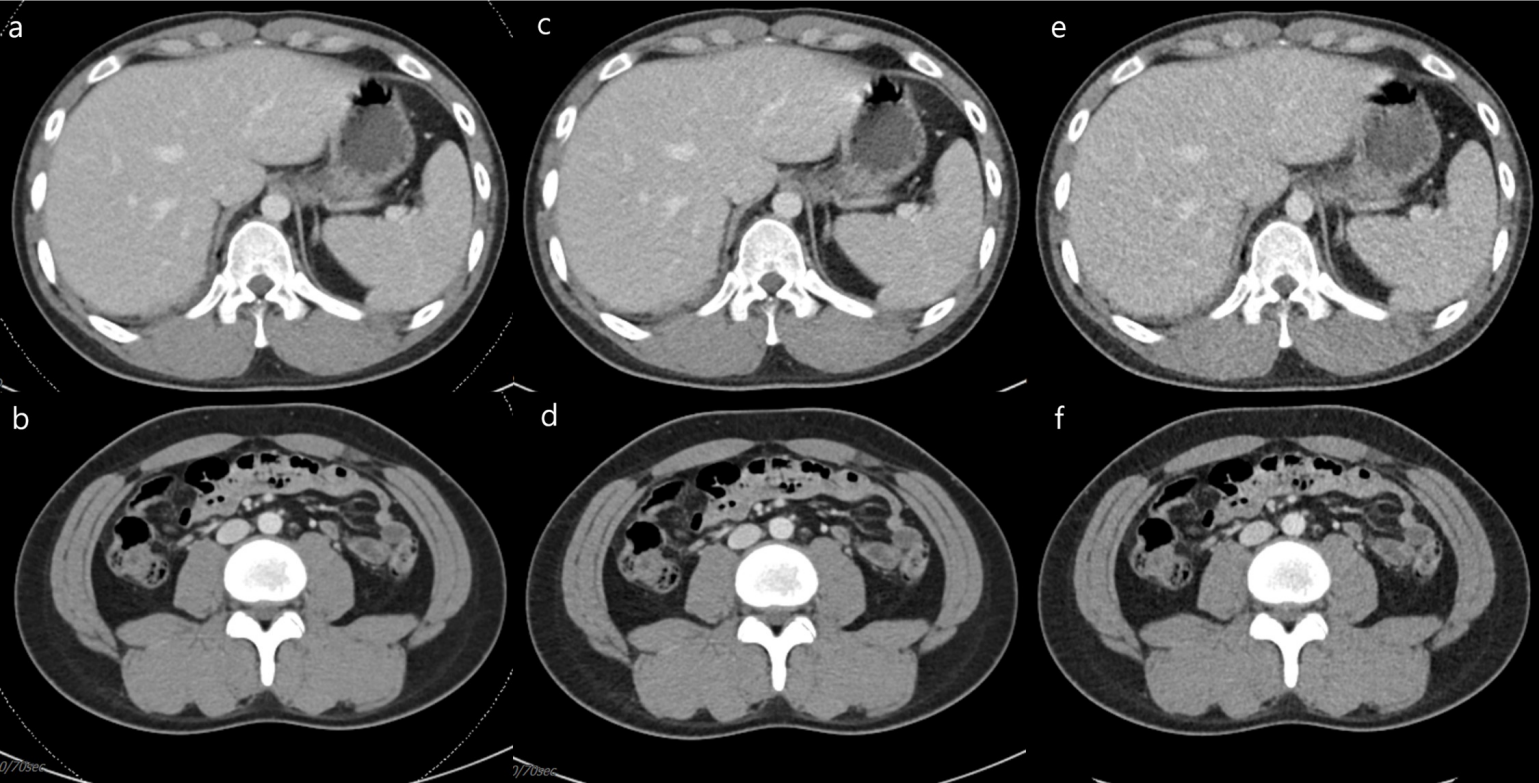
Abdominopelvic computed tomography (CT) images of a 23-year-old woman with Hodgkin’s lymphoma, complete remission state (body mass index, 24.2 kg/m^2^; effective diameter, 24.0 cm). The three different types of CT images, acquired according to the radiation dose (a-b: standard-dose CT, 5.6 mSv; c-d: low-dose CT, 3.7 mSv; e-f: ultralow-dose CT, 1.8 mSv), show the absence of enlarged lymph nodes and splenomegaly. Identical reports of the aforementioned features were also obtained from two radiologists. The overall image quality score was 4 for the ultralow-dose CT images and 5 for the low-dose and standard-dose CT images, according to three readers (two radiologists and one hematologist).
